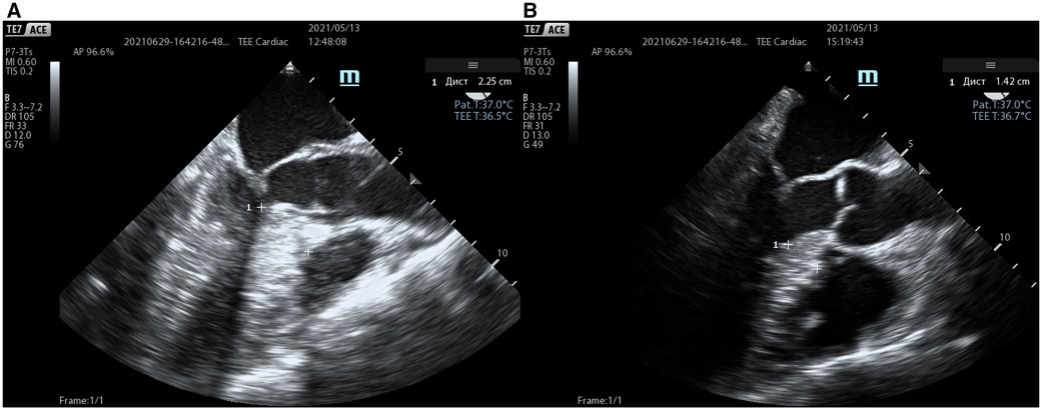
Intraoperative TEE images (mid-oesophageal aortic valve long-axis view) in early systole before (A) and after (B) procedure; elimination of the left ventricular outflow tract obstruction with concomitant procedures on the mitral valve subvalvular apparatus. Septal thickness (1) was measured before and after correction. TEE: transoesophageal echocardiography.
